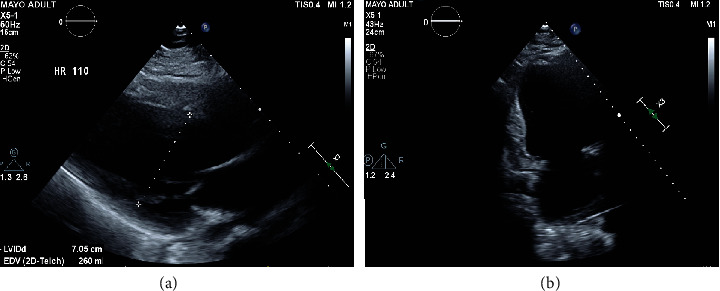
Transthoracic echocardiogram images in the (a) parasternal long axis and (b) apical 2-chamber views demonstrating profound left ventricular dilatation, as well as left atrial enlargement.
